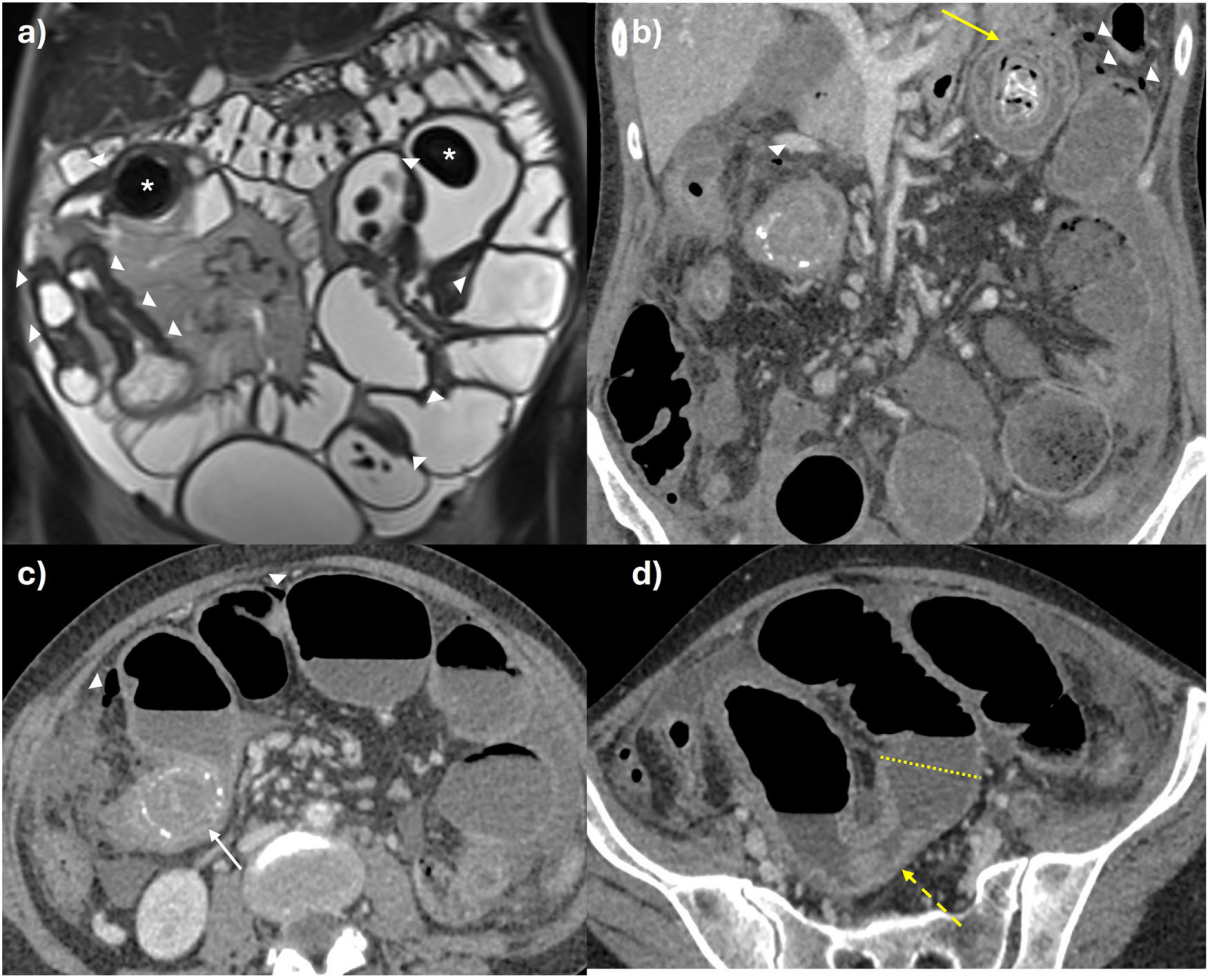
53-year-old female patient with chronic abdominal pain. a Coronal T2-weighted MR Enterography image shows multifocal stricturing disease involving most of the small bowel. Strictures (arrowheads) are mostly long (> 3 cm), and there is associated bowel dilation and multiple enteroliths (asterisk on larger examples), indicating chronic obstruction. The strictures are mainly chronic; mostly low T2 signal, maintained on the fat-suppressed sequences, not shown. There is mild superadded activity as shown by the mesenteric free fluid. CT study 2 months later when the patient presented acutely with severe, generalised abdominal pain. b Coronal, (c) axial images show locules of free gas (arrowheads) and increased free fluid due to perforation from one of the proximal tight strictures with impacted enteroliths (arrow). d Axial image shows a deep pelvic stricture (dashed arrow) with mural thickening and hyperenhancement, and dilated bowel loops
